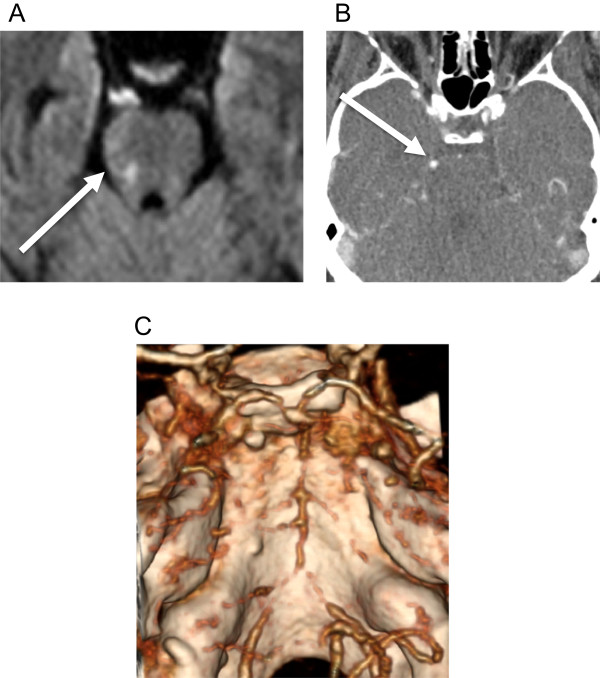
Right pons ischemia. A. Diffusion weighted MRI showing restriction in right pons (arrow) consistent with ischemia on October 29, 2012. B. Contrast CT with right superior cerebellar artery (arrow) enhancement. C. Reformatted 3-D CT angiography on Oct 29, 2012 showing diffuse vasculopathy at the basilar arteries and bilateral vertebral arteries.
